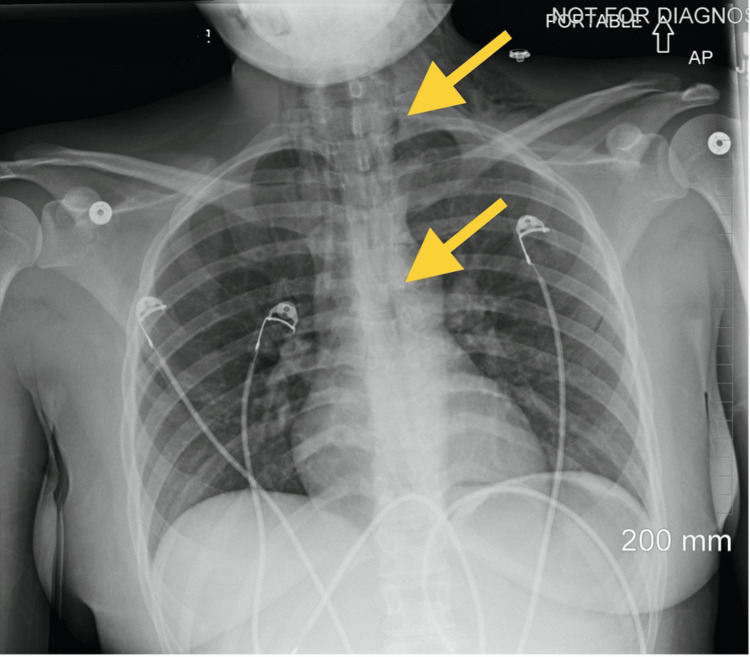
Chest X-ray showing pneumomediastinum and subcutaneous emphysema at the cervicothoracic junction (arrows)
